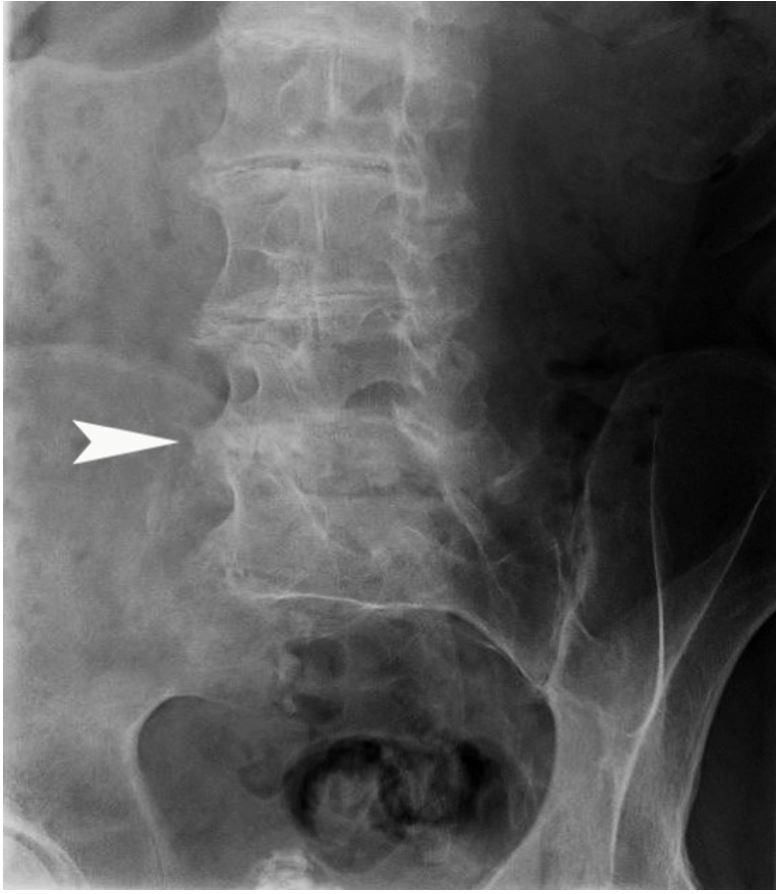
Radiographic findings in alkaptonuria. X-Ray radiography of lumbar spine showing extensive lumbar disc degeneration with disproportionately minor osteophytes and calcifications.
